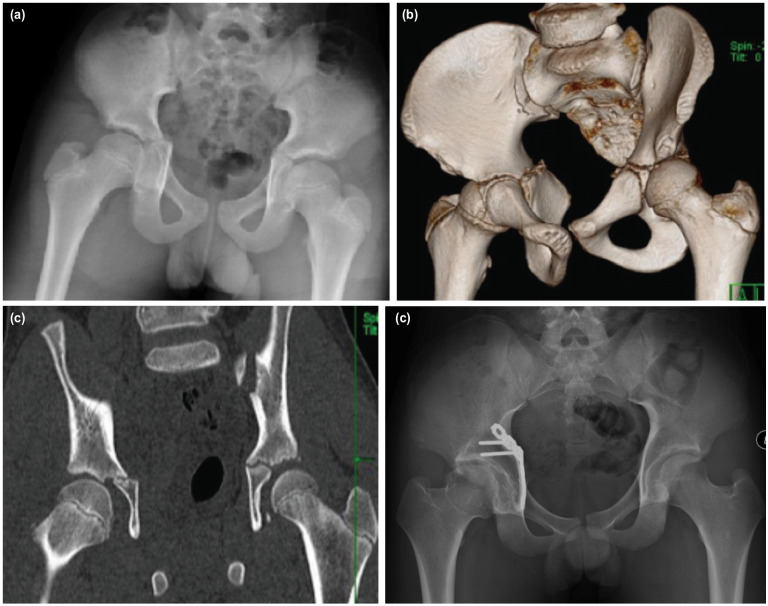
A 13-year-old boy pillion rider sustained a displaced trans-physeal fracture over right acetabulum. (a) Initial pelvis AP radiographs shows a displaced transphyseal acetabular fracture. (b) 3D CT image of pelvis depicted the acetabular physeal involvement of fracture. (c) AP image of CT scan. (d) Stabilisation was done using buttress quadrilateral plate with screws avoiding the acetabular physis. (e) There is evidence of femoral head avascular necrosis and subsequently well-remodelled six years after injury (e). Both modified Merle d'Aubigné-Postel and HHS functional outcome are excellent.
